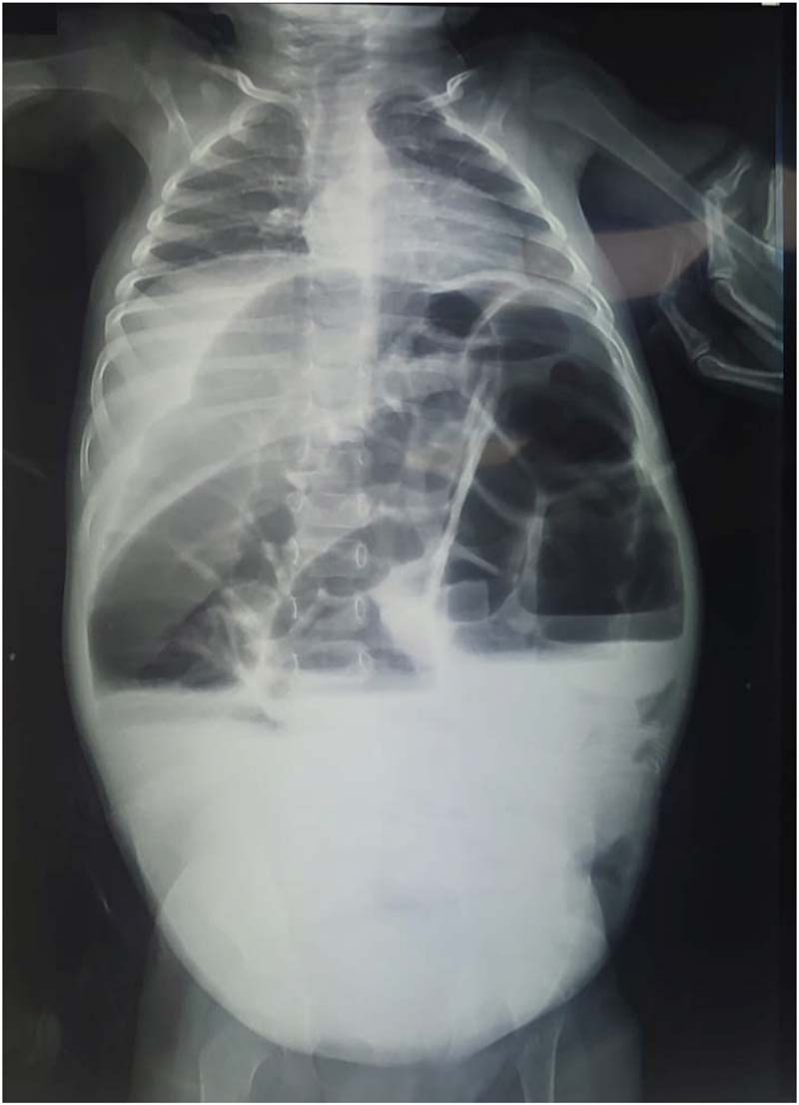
X-ray image showing intestinal-type hydroaeric levels and gastric stasis.
